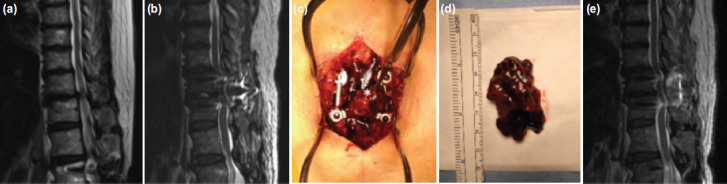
Case 1; (a) MRI showing T11/T12 stenosis due to degenerative disc disease and facet arthropathy. (b) MRI showing spinal epidural haematoma with spinal cord compression; (c,d) intra-operative photo showing spinal epidural haematoma (white asterisk in c); and (e) MRI after evacuation of haematoma showing complete decompression of the spinal cord.
